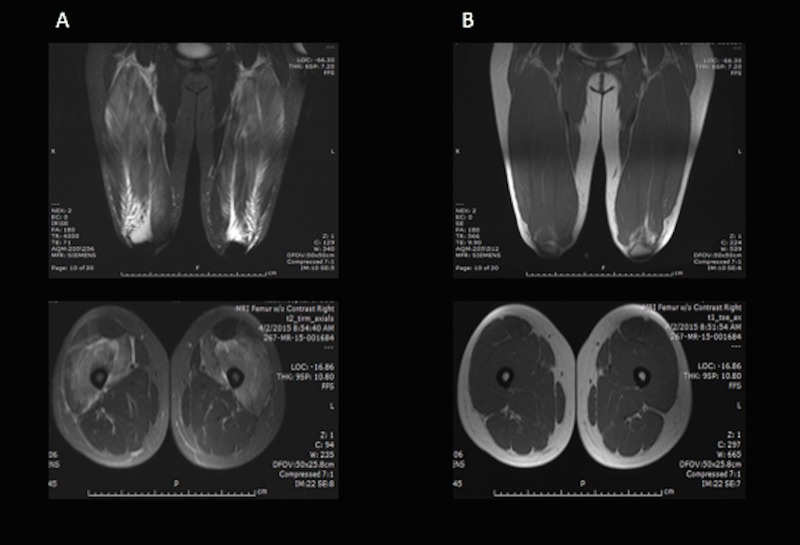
MRI Images of the Thigh Show Swelling in the Anterior Compartment with a Feather-like Signal within the Vastus Musculature Bilaterally A) T2-weighted MRI, B) T1-weighted MRI
